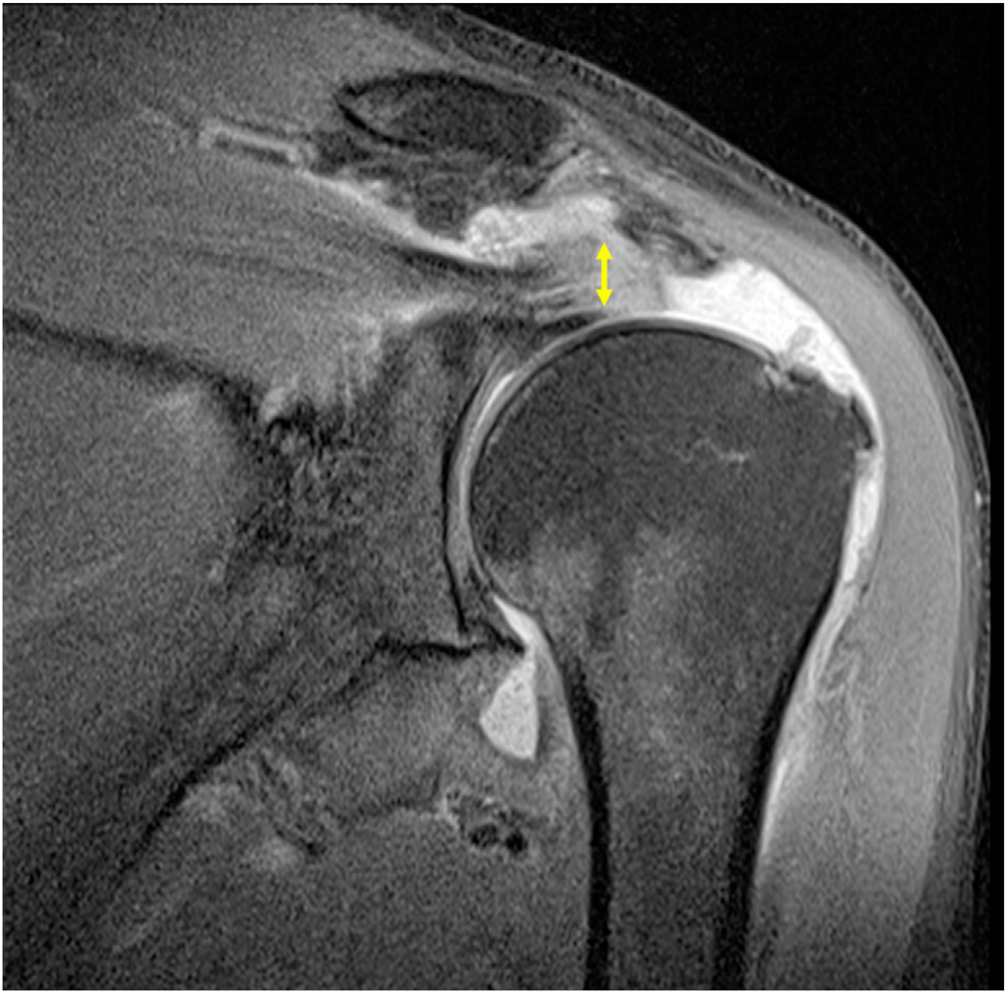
A coronal proton-density magnetic resonance imaging with fat saturation of a left shoulder shows a retracted supraspinatus tear with an abnormally thickened tendon stump (10 mm, yellow arrow), consistent with a possible Fosbury flop tear.
